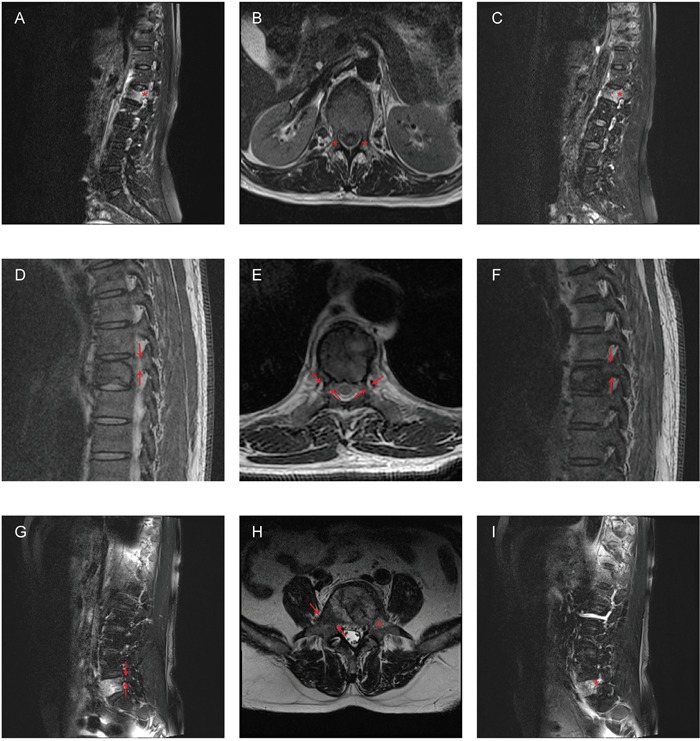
Examples of misdiagnosis according to MRI images A-C. A 58-year-old woman with L1 metastases from breast cancer. The patient only had the left pedicle involved, whereas both pedicles appeared involved in the sagittal (A, C) and transversal (B) MRI images. D-F. A 54-year-old man with L1 metastases from renal cell carcinoma. The patient had the left pedicle involved, whereas both pedicles appeared spared in the sagittal (D, F) and transversal (E) MRI images. G-I. A 67-year-old man with L5 metastases from lung cancer. The patient had both pedicles involved, whereas only the left pedicle appeared involved in the sagittal (G, I) and transversal (H) MRI images. Arrows: pedicles with normal signal in MRI; Asterisks: pedicles with abnormal signal in MRI.
